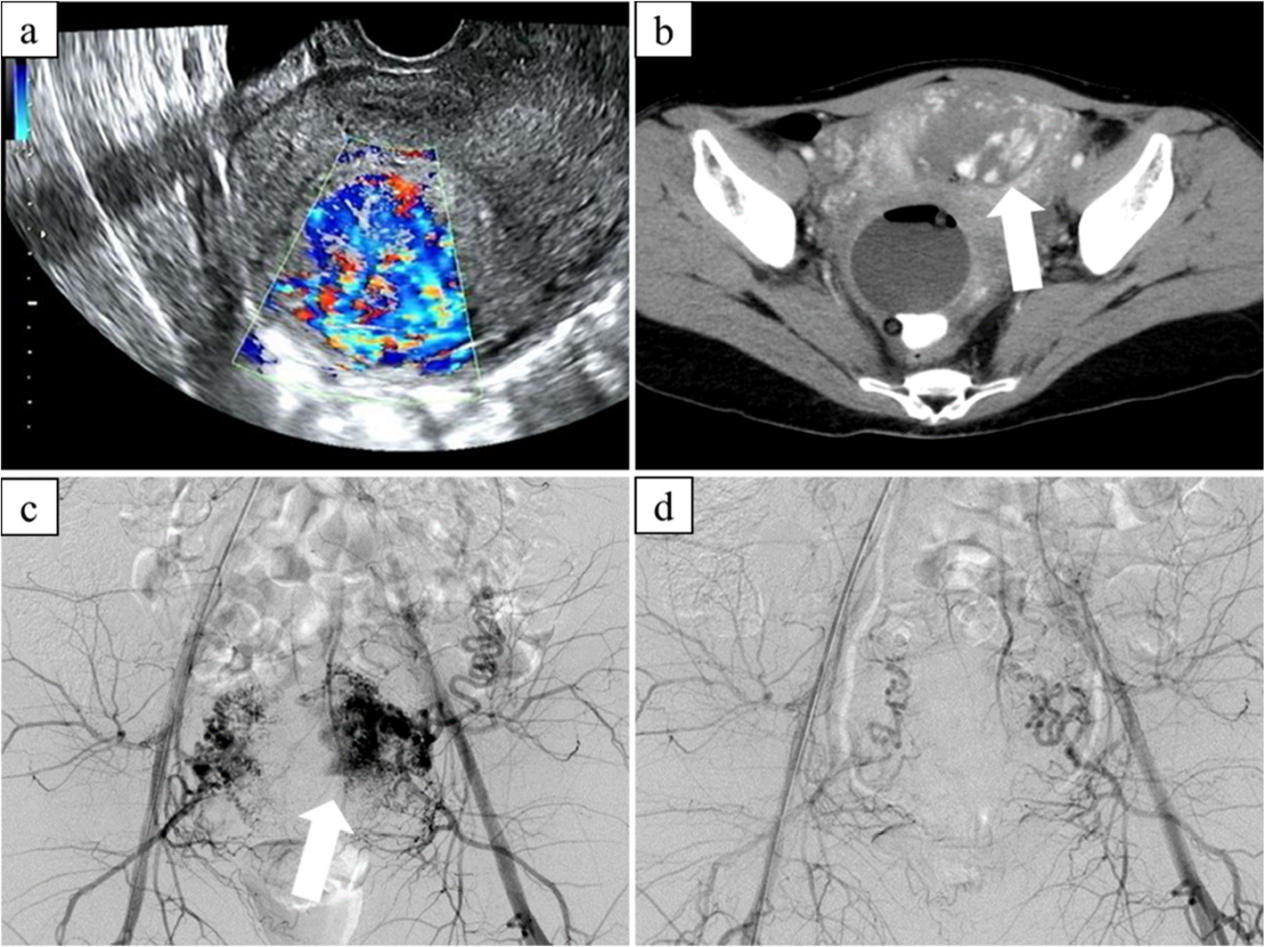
31-year-old patient with vaginal bleeding after vaginal delivery. a Transvaginal ultrasonography showing a hypervascular hyperechoic mass in the endometrium. b Arterial-phase contrast enhanced CT showing focal enhancement (arrow) within the endometrium. c Aortography showing numerous spiral arteries and a focal blush in the left side of uterus (arrow). d Post-embolization aortography showing contrast stasis without focal blush
